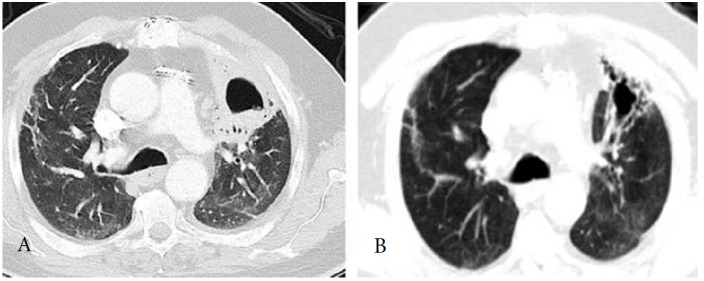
a: Chest CT of the patient who presented with a cavity in the left upper lobe at 12 weeks post-COVID. b: CT showed a regression of the cavity after 6 weeks of antibiotic treatment. (From archives of Aydın Yılmaz)
